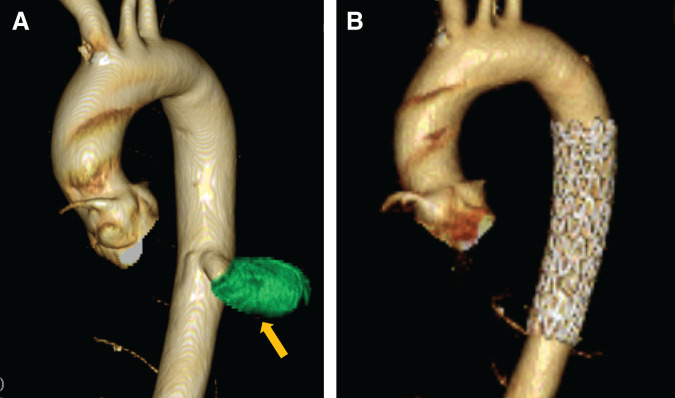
3D CT angiography performed before and after TEVAR. (A) This figure shows an irregular aneurysm arising from the descending aorta (arrow). (B) This figure demonstrates successful flow exclusion within the aberrant artery and its aneurysm. TEVAR, thoracic endovascular aortic repair
